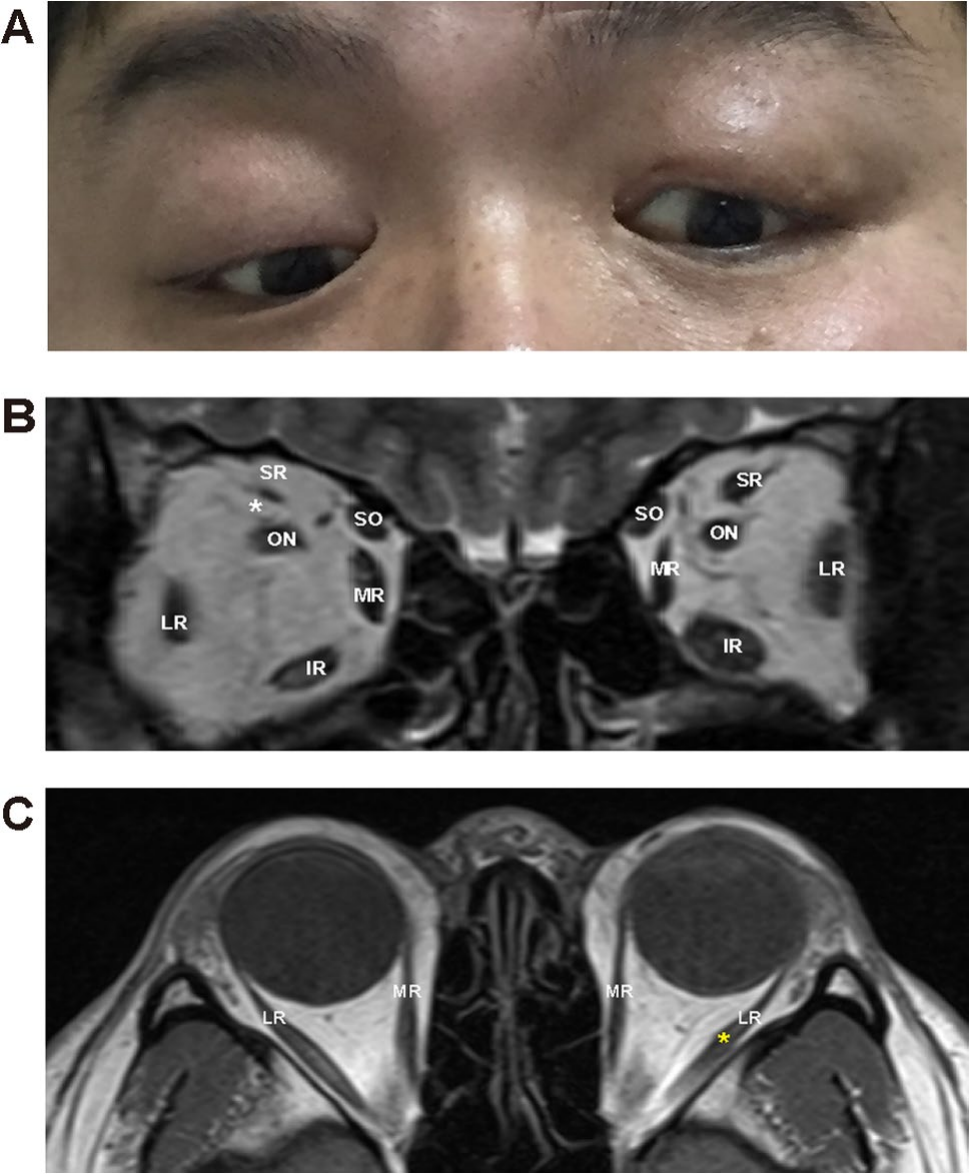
The eye position and MRI in Subject 02. A Eye position demonstrated ptosis, esotropia, and hypoplasia. B Coronal MRI showed atrophy of the left SR muscle (white asterisk). C Axial MRI showed the LR muscle of the left eye had a string-like configuration, which suggested muscle fibrosis the hypoplasia (yellow asterisk). MRI, magnetic resonance imaging; SR, superior rectus. LR, lateral rectus. MR, medial rectus. IR, inferior rectus. SO, superior oblique. ON, optic nerve
